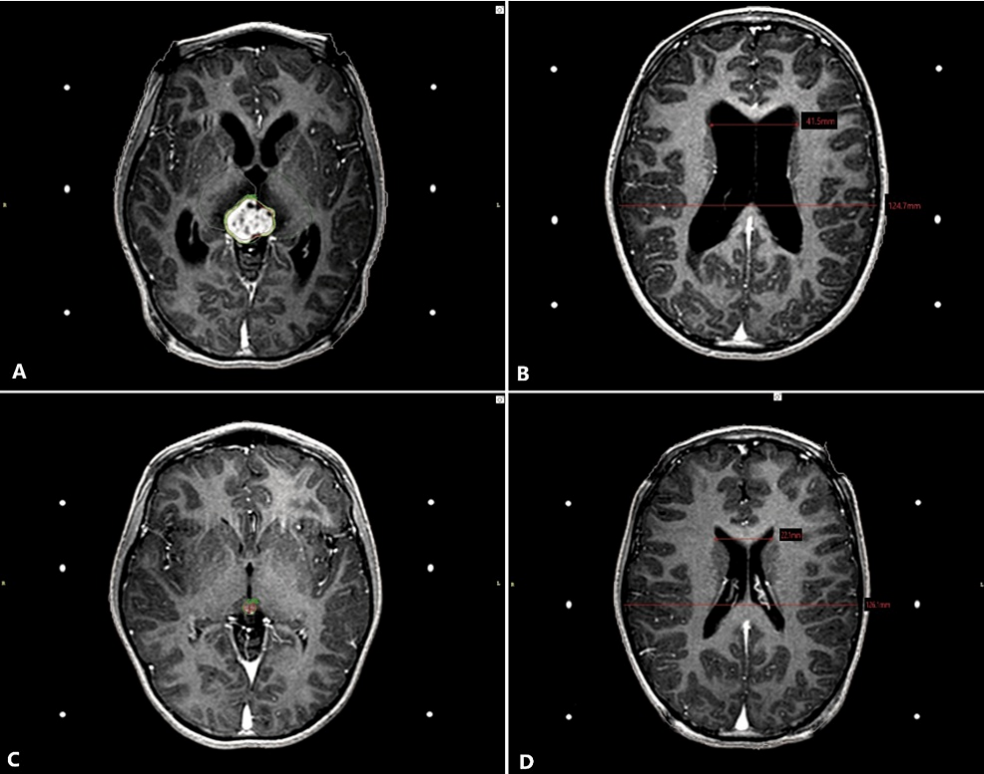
Adaptive SRS plan for patient three with pineocytoma in mesencephalic tectum causing hydrocephalus A. The first session of the adaptive SRS plan to treat a pineocytoma in the mesencephalic tectum. The plan included a GTV of six cubic centimeters (in red) with a prescription of 13 Gy with a 50% isodose line covering (in light green). The cerebral peduncles were drawn as OAR and limited the dose prescription. B. During the first session, the lateral ventricles showed an EVAN’S index of 0.33. C. After 42 days, the axial T1 gadolinium-enhanced MRI of the second session showed a reduction of the tumor in the mesencephalic tectum. The GTV (in red) included in this plan was 0.1cc and it was treated with 10 Gy with the 50% isodose line covering (in green). The tumor no longer compromises the quadrigeminal cistern. D. The lateral ventricles showed a reduction in their volume, and their EVAN’S index was 0.17. SRS: stereotactic radiosurgery; GTV: gross target volume; Gy: Grays; OAR: organs at risk.
